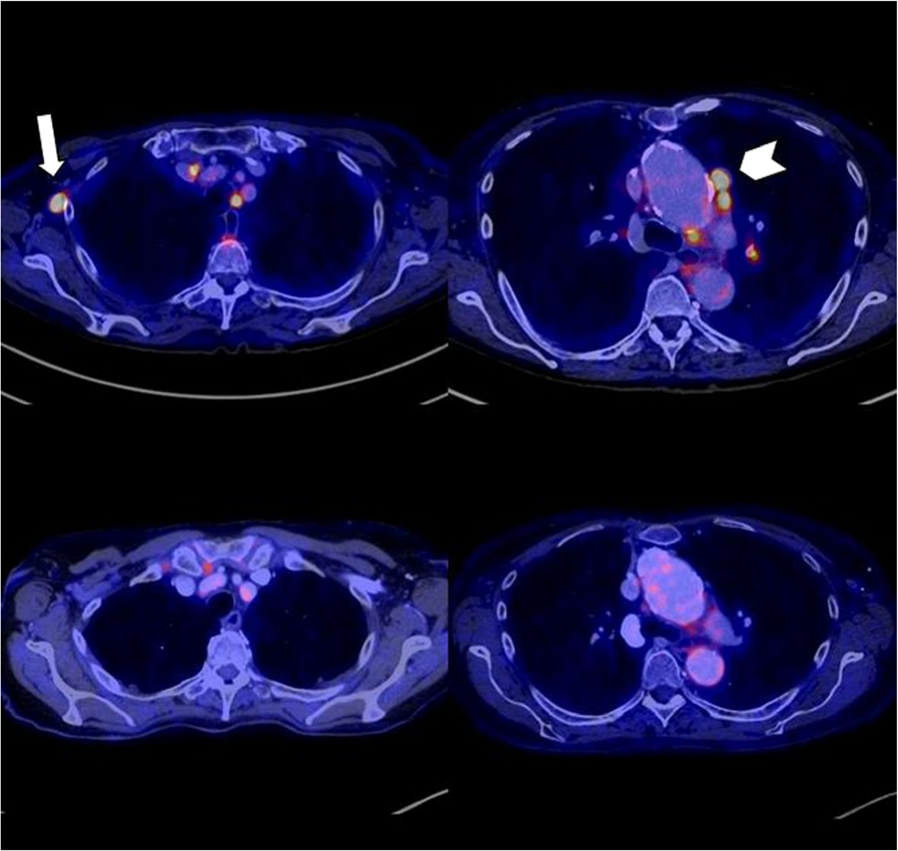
Patient 2. Upper row - before tuberculostatic therapy: high FDG uptake in several mediastinal and one right axillary (arrow) lymph nodes. Additionally, further lymph nodes with high FDG uptake, especially in the left paraaortal (arrow head) region. Lower row - 7 month follow up after initiation of tuberculostatic therapy: No FDG uptake in the right axillary lymph nodes and significant regression of FDG uptake of the paraaortal lymph nodes after therapy. All PET positive lymph nodes had a morphological correlate and showed a significant size reduction after therapy in contrast enhanced CT (not shown).
